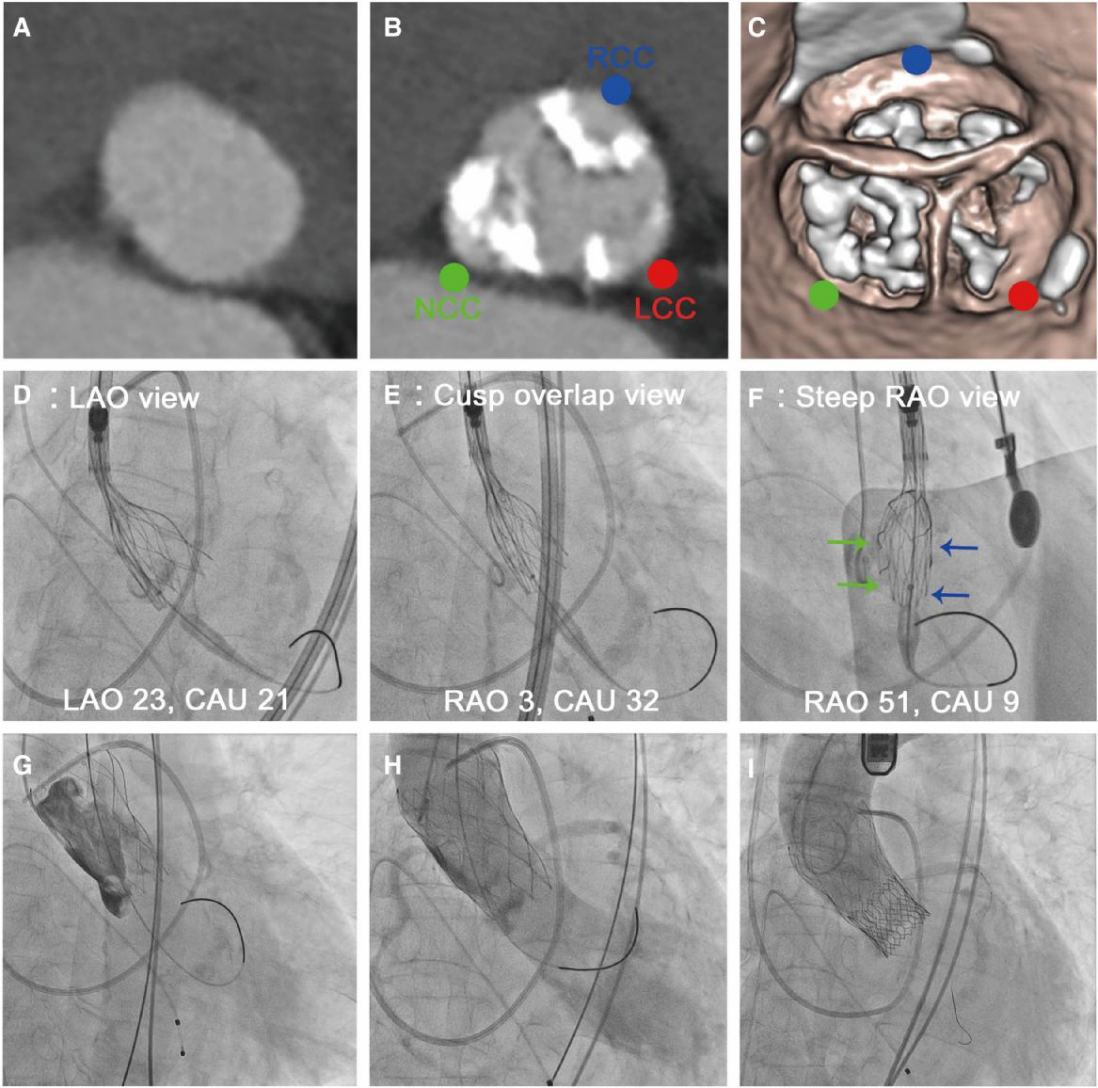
Significant transcatheter heart valve under-expansion leading to embolization. (*A–C*) Pre-procedural computed tomography imaging showing a heavily calcified aortic valve, especially in the right- and non-coronary cusps. (*A*) Aortic annulus. (*B*) 6 mm above the annulus. (*C*) Volume-rendering image. (*D* and *E*) In the left anterior oblique and cusp-overlap views, the transcatheter heart valve seemed to be well expanded. (*F*) Only the steep right anterior oblique view elucidated a constrained transcatheter heart valve at 80% deployment. Significant transcatheter heart valve under-expansion is indicated by green arrows in the non-coronary cusp and blue arrows in the right-coronary cusp. (*G*) Transcatheter heart valve embolization just after the final release. (*H*) Severe aortic regurgitation. (*I*) Emergent implantation of a balloon-expandable valve proved successful as a bailout. The blue circle represents the right-coronary cusp; the red circle represents the left-coronary cusp; and the green circle indicates the non-coronary cusp.
